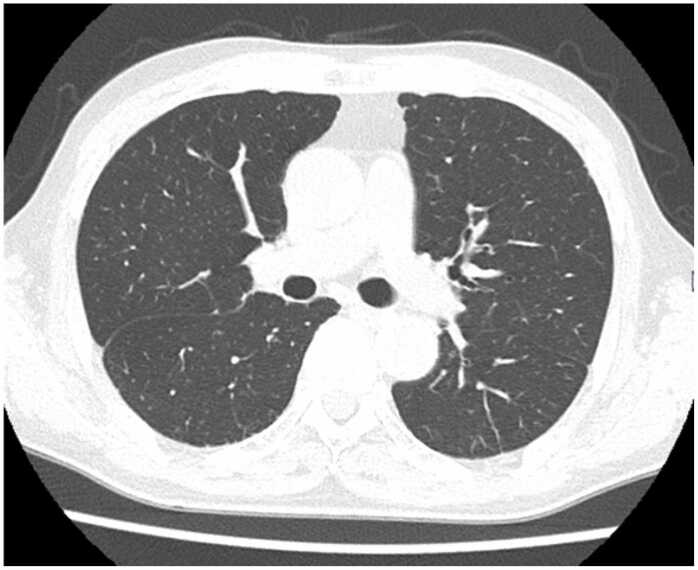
Initial chest X-ray and chest computed tomography (CT) at the time of diagnosis.
